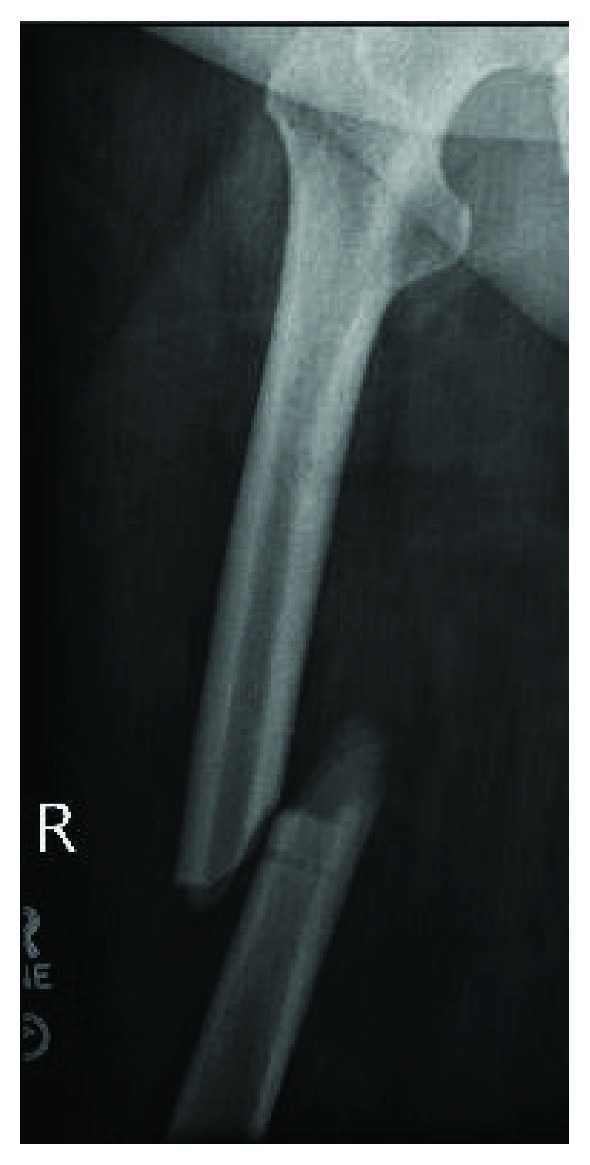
Right femur radiograph with a unicortical tracking pin site clearly visible.
